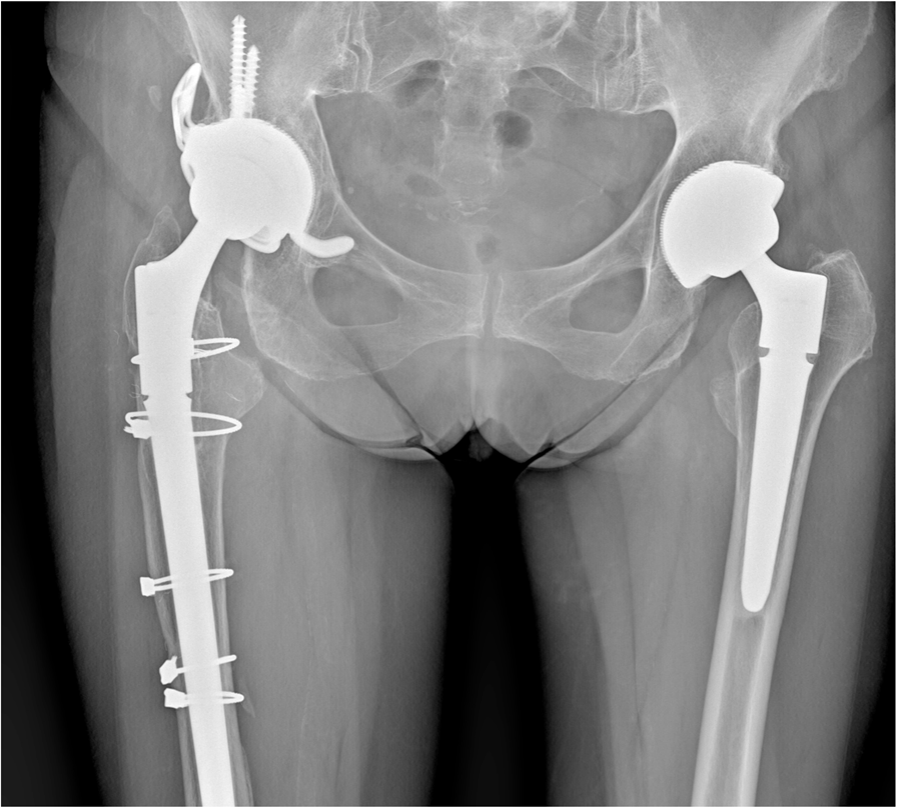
X-ray at 7 years’ follow-up after revision surgery
